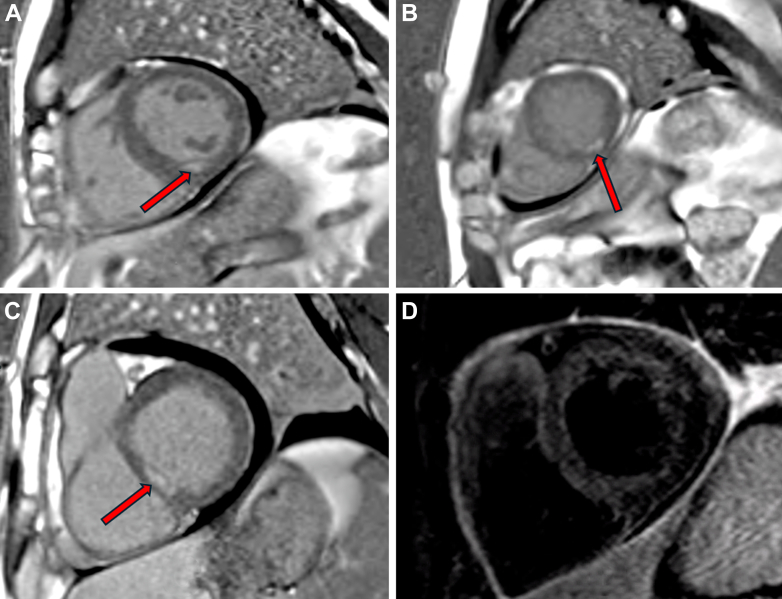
Cardiac Magnetic Resonance Imaging Findings Short-axis late gadolinium enhancement (LGE) images show patchy mid-myocardial enhancement in multiple left ventricular segments (red arrows): (A) mid-inferior wall at the papillary muscle level; (B) apical inferolateral wall; and (C) basal interventricular septum, with a non-subendocardial, noncoronary distribution. (D) Dark-blood T2-weighted short-axis (STIR) imaging shows increased T2 signal in the anteroseptal and anterior walls, consistent with myocardial edema. Combined LGE and T2 findings indicate active myocardial inflammation with associated edema.
